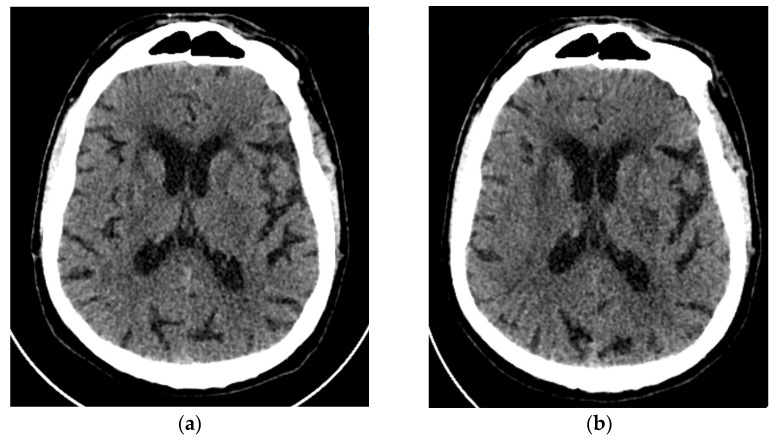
Axial brain CT scan (a) on an emergency basis with ischemic microangiopathy, without any obvious acute ischemic stroke or hemorrhage; (b) an axial CT scan 2 days later with a hypodense lesion in the left basal ganglia region, on the distribution of the left anterior choroidal artery (AChA) and ischemic microangiopathy periventricular and on the basal ganglia.
